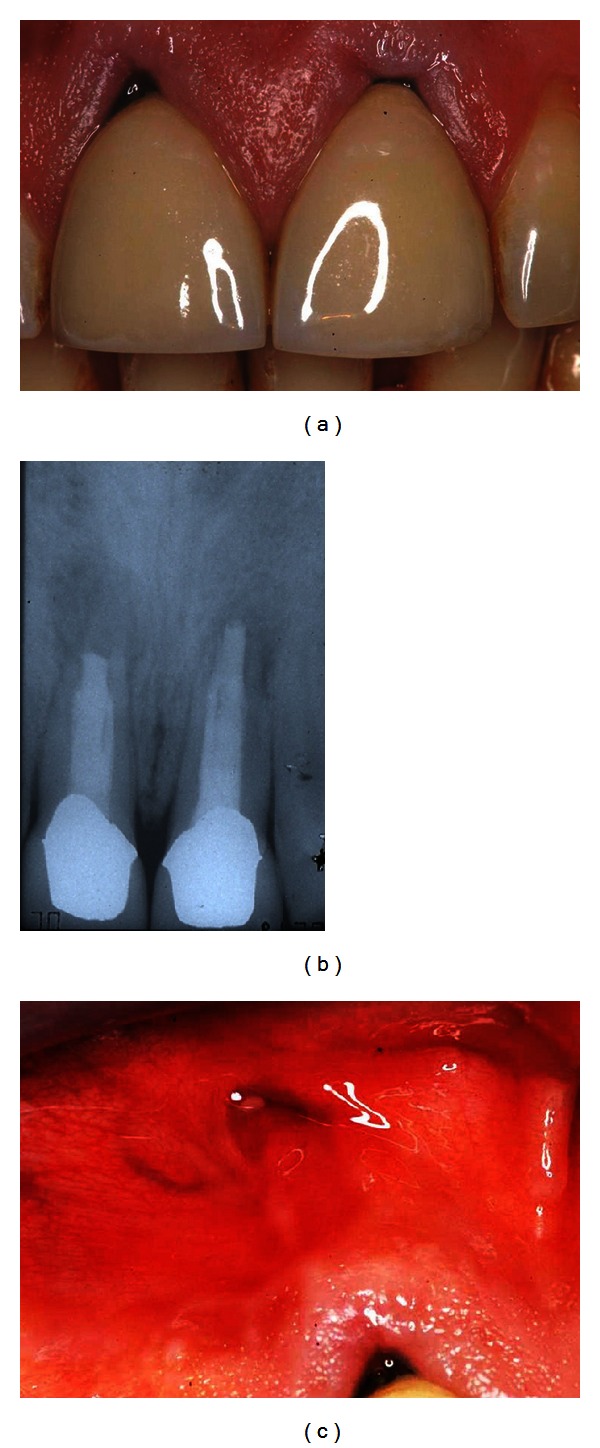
Radiographic (a) and clinical control (b) with the presence of a secreting fistula in correspondence with the mucosa in the apical area of 1.1 (c).
